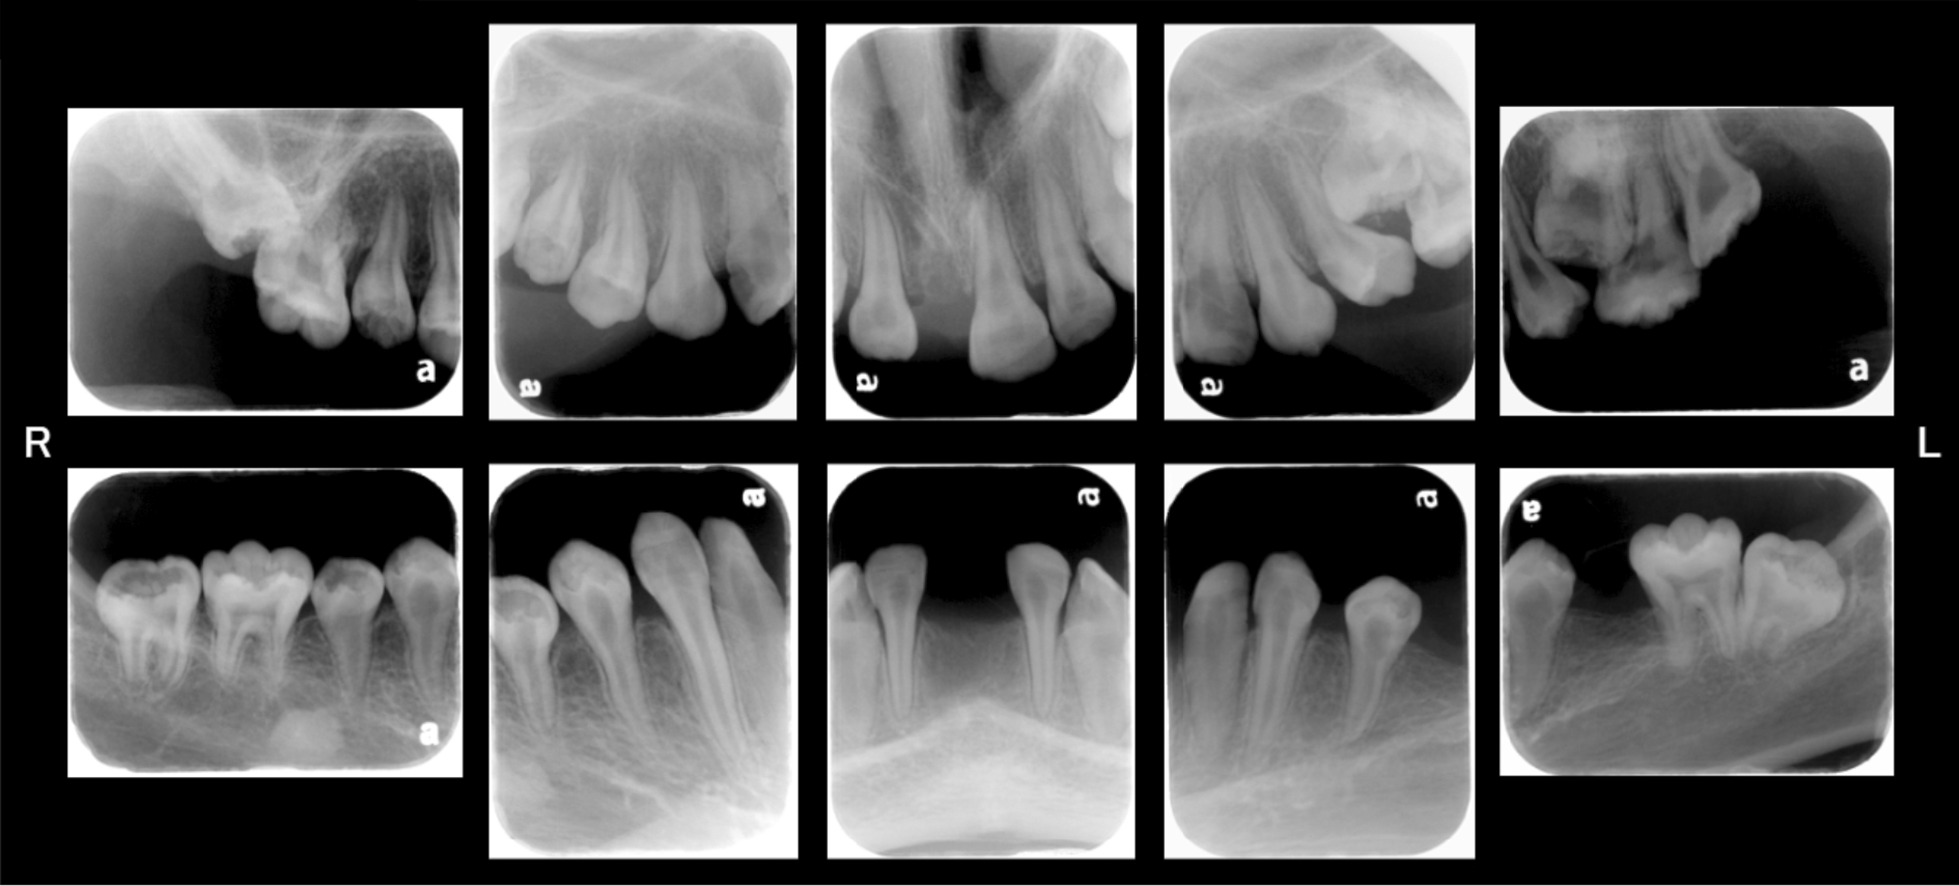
Periapical radiograph images obtained at 14Y9M of age
